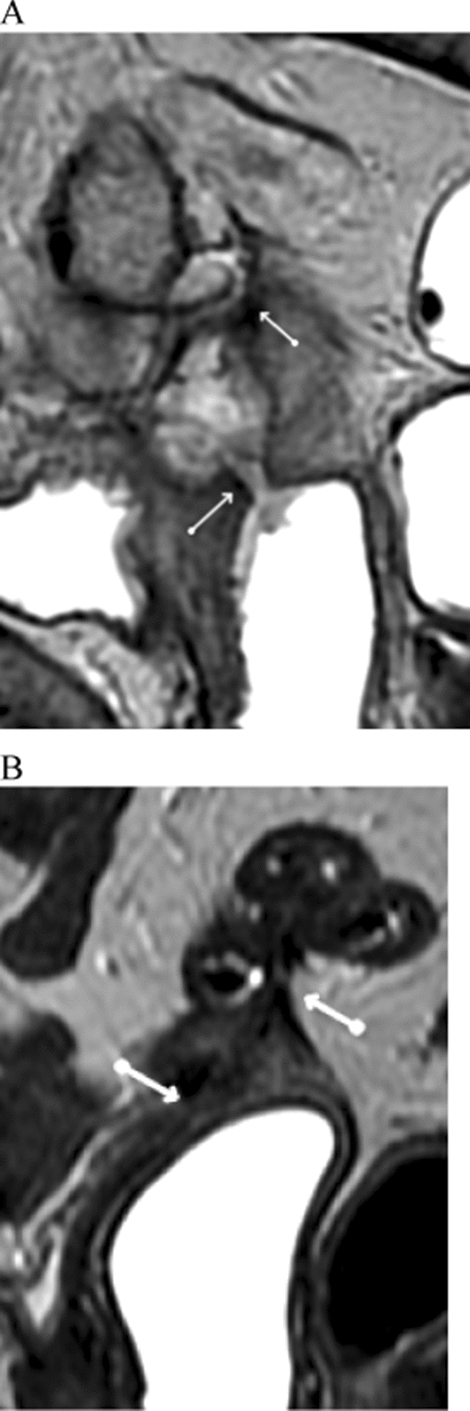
(Patient 12)—Sagittal T2-weighted magnetic resonance image shows fistulous track (white arrows) communicating the upper third of the vagina with abscesses in the pelvic cavity and intestinal loops (A). Magnetic resonance image after laser therapy no longer identifies the fistulous track with presence of low signal intensity tissue on T2-weighted images (white arrows), inferring fibrosis (B)
